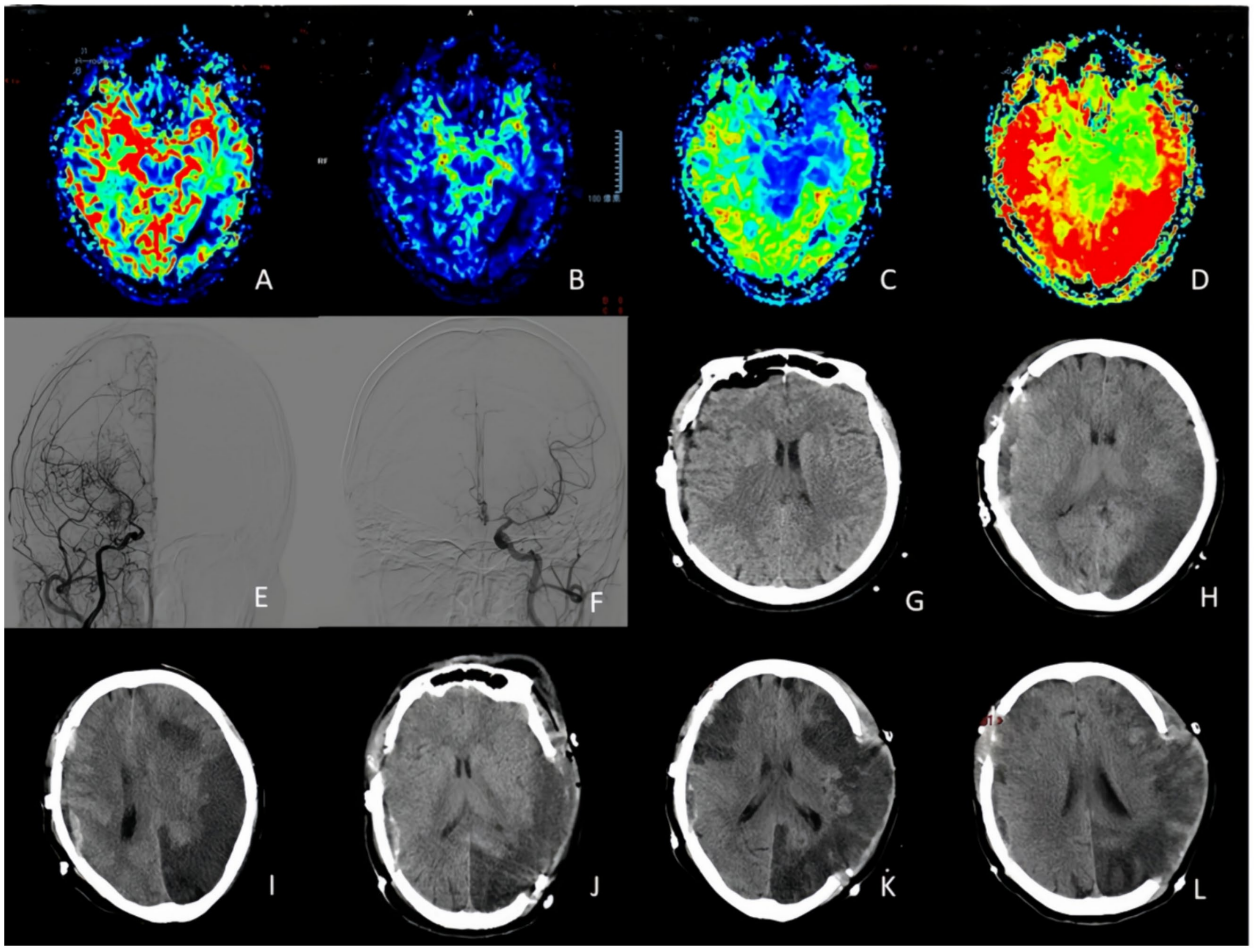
A 63-years-old woman who experienced right side lower extremity weakness one prior surgical treatment. (A,B) PWI indicated CBV decreased in the left temporal occipital lobe and right frontal and parietal lobe, and CBF decreased in the right cerebral hemisphere and left temporal and occipital lobe. (C,D) PWI showed MTT and TTP delayed in right cerebral hemisphere and left temporal occipito-parietal lobe. (E,F) DSA suggested occlusion of the right internal carotid artery with moyamoya vessels. Left middle cerebral artery and anterior cerebral artery stenosis. (G–I) CT changes on postoperative day, postoperative day 1, and postoperative day 3 indicated a severe cerebral infarction on the non-operative side (stenotic side). (J–L) On the third day, the patient underwent left side decompressive craniectomy, and CT findings were obtained at postoperative day 1, 3 and 10.
